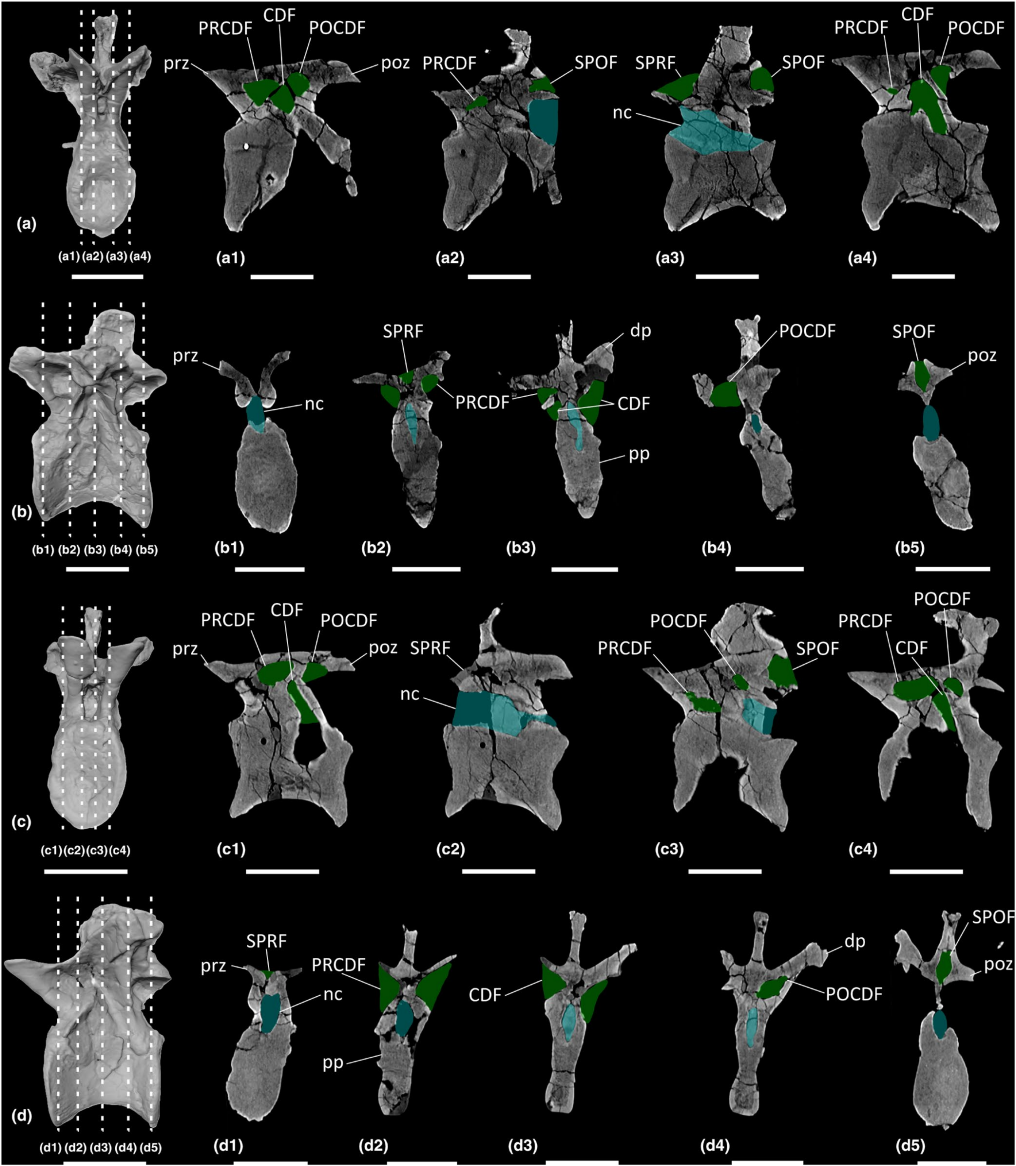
CT scan data of *Plateosaurus longiceps* MB.R.4430 ‘Skeleton C’ anterior dorsal vertebrae. (a, b) dorsal vertebra 2 MB.R.4430.23, (c, d) dorsal vertebra 4 MB.R.4430.25; (a, c) surface scan anterior view, (b, d) surface scan lateral view; (a1–a4, c1–c4) CT scan slices in parasagittal cross‐section, (b1–b5, d1–d5) CT scan slices in transverse cross‐section. CDF, centrodiapophyseal fossa; dp, diapophysis; nc, neural canal; POCDF, postzygapophyseal centrodiapophyseal fossa; poz, postzygapophysis; pp, parapophysis; PRCDF, prezygapophyseal centrodiapophyseal fossa; prz, prezygapophysis; SPOF, spinopostzygapophyseal fossa; SPRF, spinoprezygapophyseal fossa. Key: Blue highlight, neural canal; green highlight, fossa. Scale bars = 5 cm.
